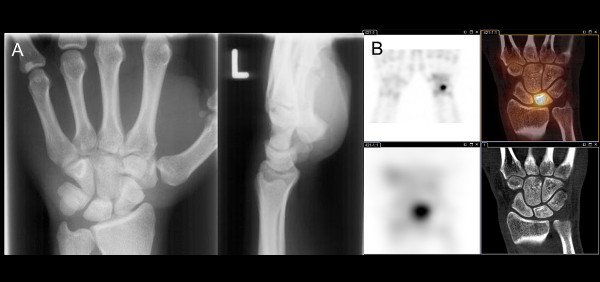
A 25-year-old male with left-sided wrist pain when grabbing and lifting. Tendinitis was suspected. (A) Plain radiographs showed no bone lesion. (B) SPECT/CT revealed an intense radioisotope uptake in the lunate bone corresponding with osteomalacia of the lunate bone followed by surgery (top row: three-dimensional (3D)-SPECT and fusion SPECT/CT, bottom row: planar SPECT and CT-alone).
